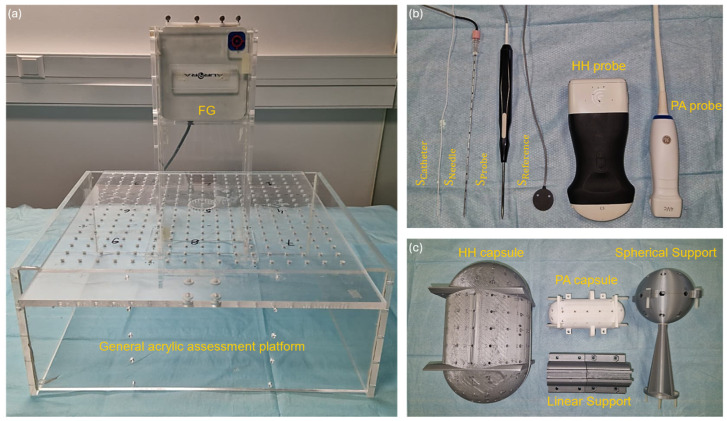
Experimental setup and equipment used for this study. (a) General acrylic assessment platform equipped with the Aurora field generator mounted on top, providing the basis for all experiments. (b) Electromagnetic (EM) sensors and ultrasound (US) probes: catheter sensor ($S_{Catheter}$, 5-DOF), needle sensor ($S_{Needle}$, 5-DOF), probe sensor ($S_{Probe}$, 6-DOF), reference sensor ($S_{Reference}$, 6-DOF), handheld US probe (Clarius C3 HD Scanner), and a 3D/4D PA probe (GE 4VC-D). (c) Custom 3D-printed probe support structures: HH capsule and PA capsule for Study 1, Linear support for Study 2, and spherical support for Study 3.
